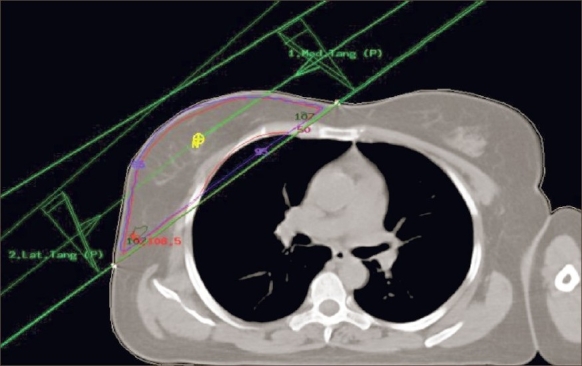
CT-based planning for a target volume of 629 cc
